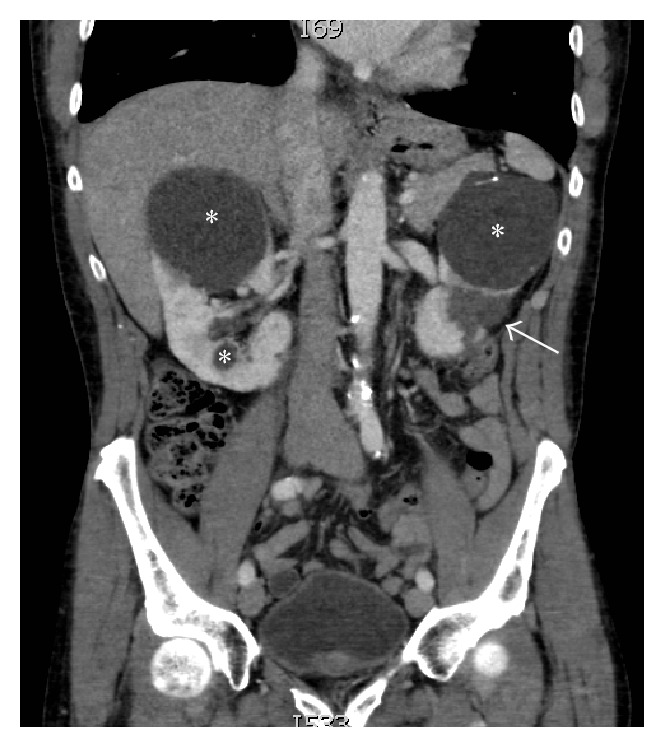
CT of the abdomen and pelvis with contrast, demonstrating a wedge-shaped area of hypoenhancement in the mid to lower pole of the left kidney (arrow), consistent with infarct from septic embolization. Incidentally noted were the large, bilateral renal cysts (*∗*), unchanged in appearance from prior imaging.
